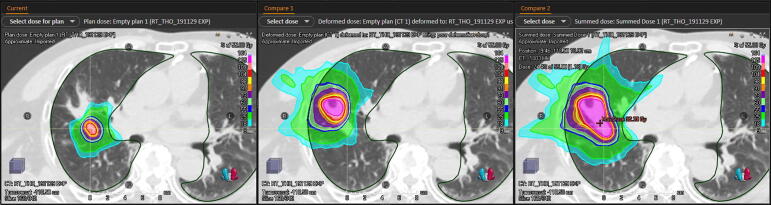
Dosimetric presentation of patient N-2. Left: current relapse treatment of 50 Gy in 5 fractions. Middle: previous SBRT irradiation dose of 55 Gy in 5 fractions registered using deformable registration on current CT. Right: cumulative dosimetry of treatments with maximal point dose of 92.4 Gy.
